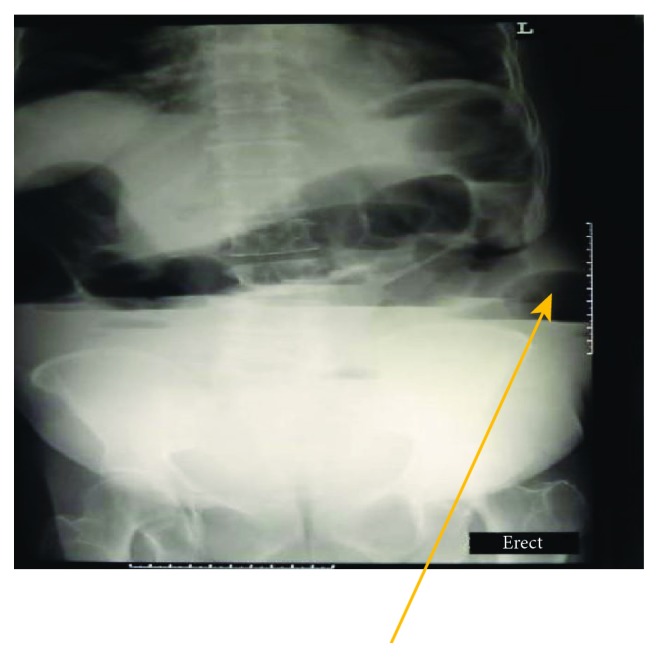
Abdominal X-ray anteroposterior showing air fluid level with a protrusion in the left anterior abdominal wall.
